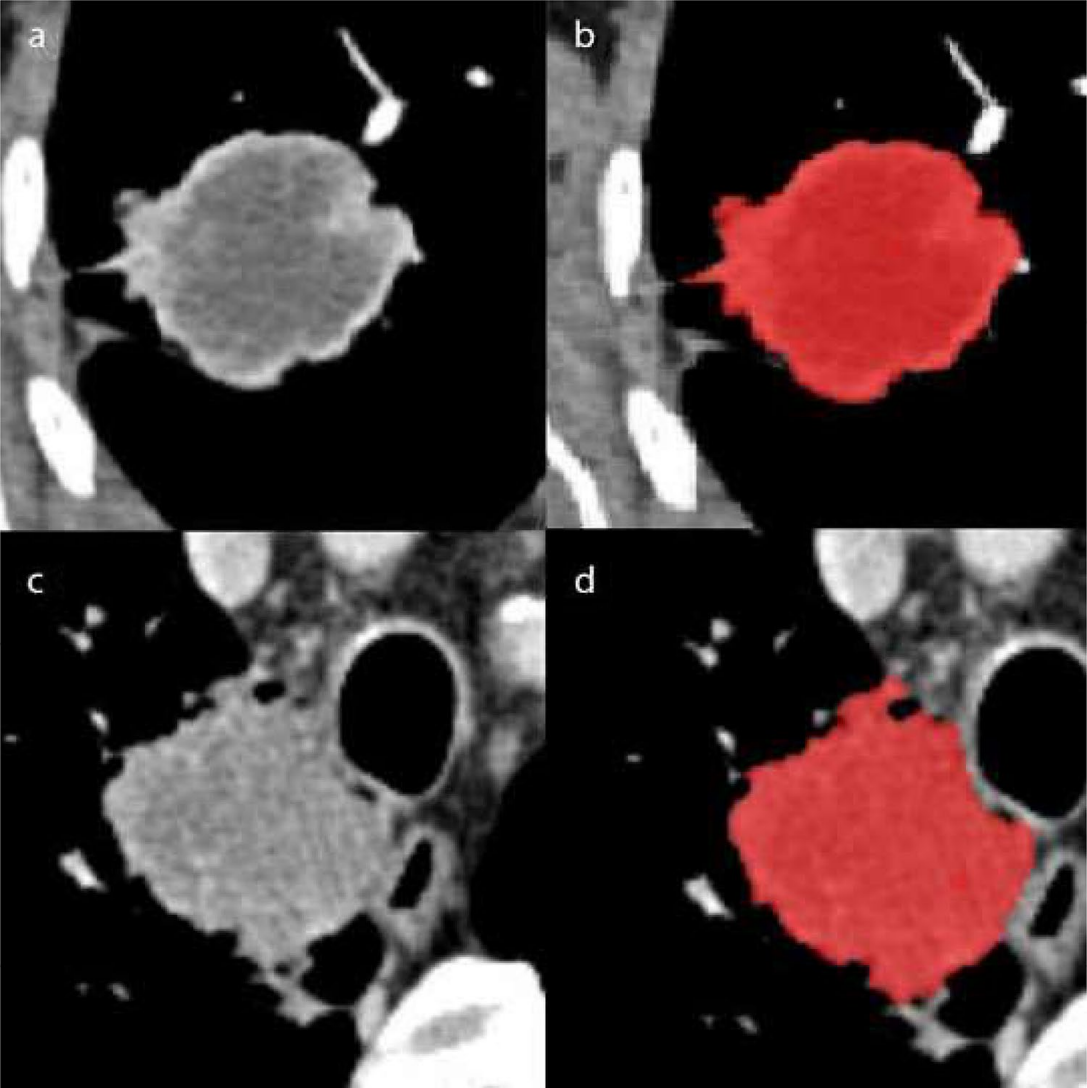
CT axial images showing two primary tumor of the lung, an ADK (a) and a SCC (c); (b) and (d) shows the same lesions contoured, respectively
